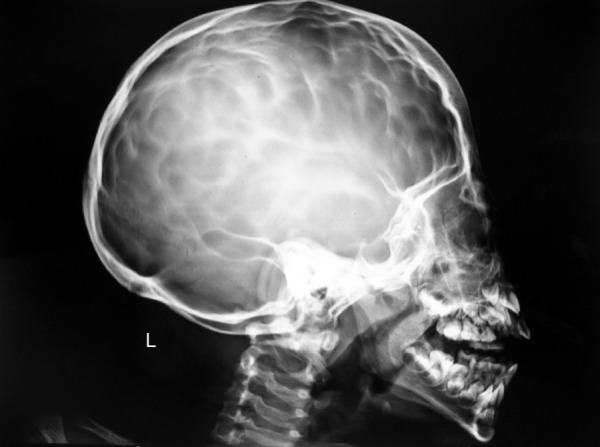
Extraoral radiograph: lateral skull view
